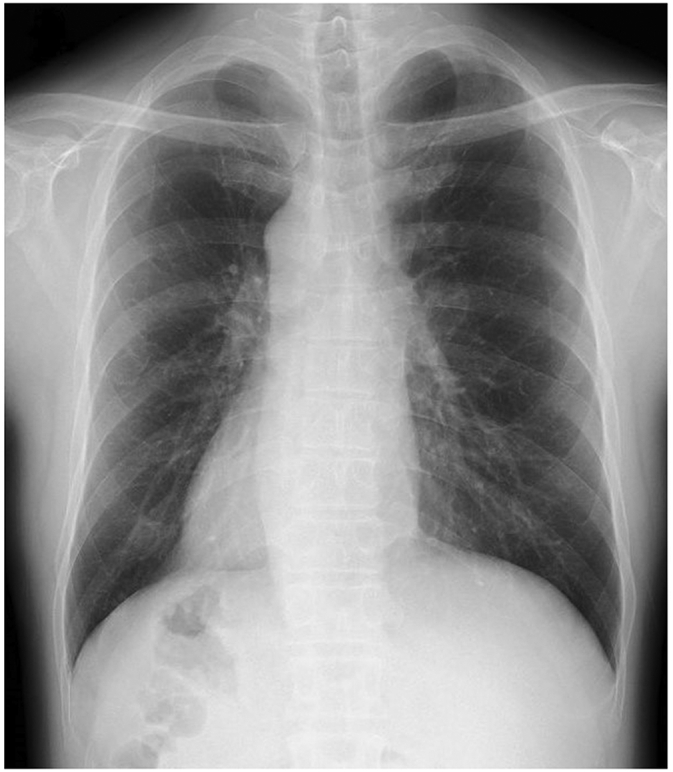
A chest X-ray showed dextrocardia.
